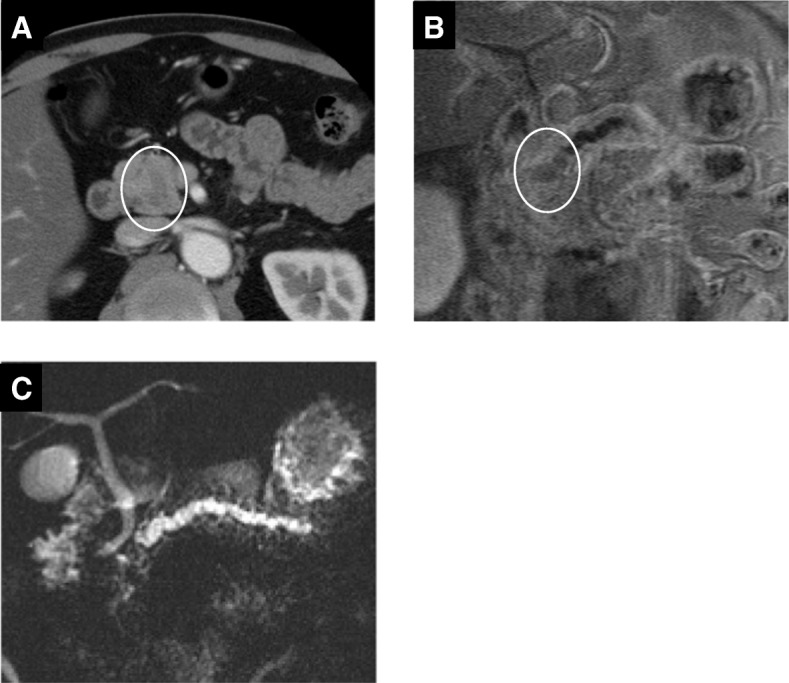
Preoperative imaging findings during the assessment of the initial resected pancreas head lesion. a Enhanced computed tomography showed a dilated main pancreatic duct (MPD) with an enhancing mass (20 mm in diameter) at the head of the pancreas (circle). b, c Magnetic resonance cholangiopancreatography showed a low-intensity area in the pancreas head (circle) and dilation of the distal side of the MPD (5 mm in diameter)
